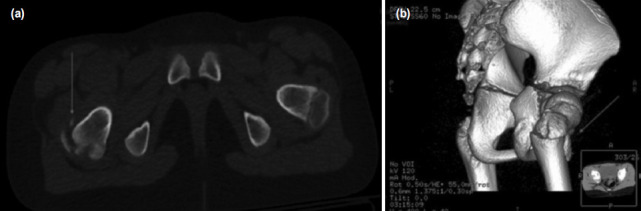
(a) Axial view (b) and 3D reconstruction CT imaging of the pelvis, made at the time of presentation, showing three-fragment fracture of the greater trochanter with the biggest fragment displaced anteriorly (marked by the arrow).
